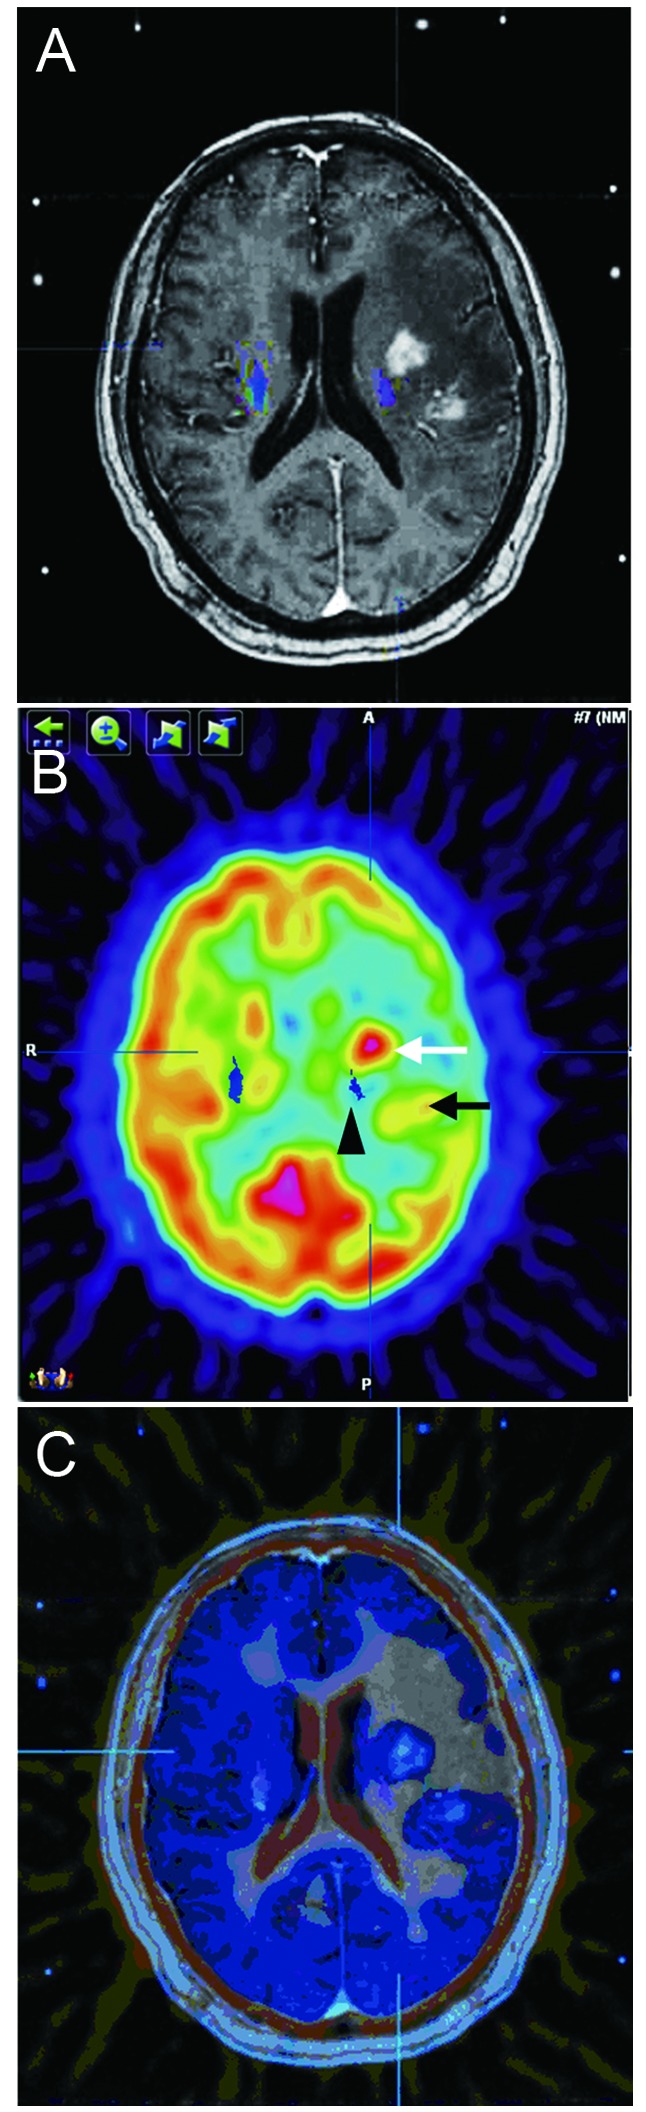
Sagittal fusion images. (A) Sagittal magnetic resonance imaging (MRI) obtained following gadolinium administration revealing two abnormal contrast-enhanced lesions located in the left basal ganglia areas. (B) Sagittal ^18^F-fluorodeoxyglucose (FDG)-positron emission tomography (PET) fusion image revealing higher FDG uptake within the upper right lesion (white arrow) than in the lower left lesion (black arrow) and the compressed left corticospinal tract of the posterior limb of the internal capsule (black arrowhead). (C) Sagittal PET-MRI fusion image demonstrating the overlap of the areas of high FDG uptake and the contrast-enhanced lesion.
